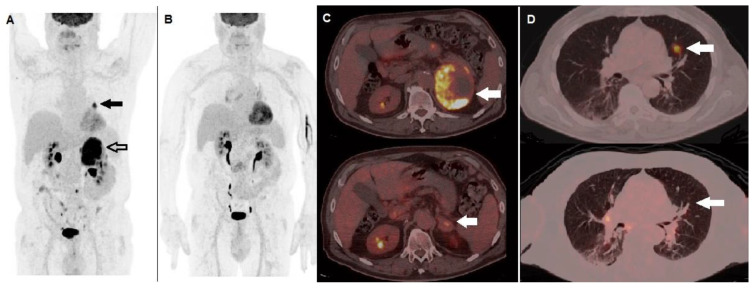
A 62-year-old male, previously submitted to excision of nodular cutaneous melanoma (Breslow thickness of 8 mm, Clark level IV, stage pT4a), performed ^18^F-FDG PET/CT for staging before therapy. (A) MIP image showed areas of increased tracer incorporation in the left lung (black arrow) and adrenal gland (black bordered arrow). Molecular analysis was positive for BRAF V600e mutation and he started combination of BRAF and MEK inhibitors (dabrafenib plus trametinib). PET/CT MIP (B) performed after 3 months showed metabolic response to therapy. Fused corresponding PET/CT axial of the abdominal region (C) demonstrated almost complete regression of the non-homogenously hypermetabolic lesion in the left adrenal gland when baseline (upper row, arrow) is compared with follow-up PET/CT scan (lower row, arrow). Fused PET/CT axial of the lung (D) demonstrated regression of the hyperactive nodule in the left lung when baseline (upper row, arrow) is compared with follow-up scan (lower row, arrow).
